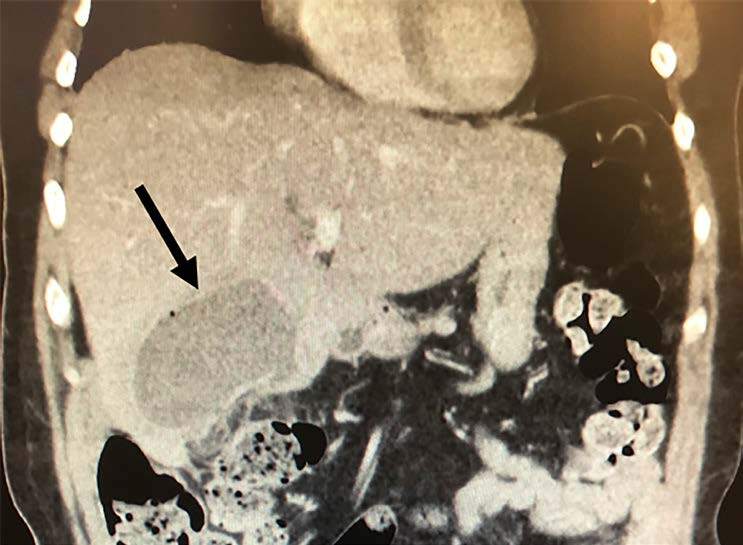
Sagittal view of computed tomography of abdomen/ pelvis with intravenous contrast demonstrating a 7.3 × 5.7 × 7.7 centimeter rim-enhancing fluid collection in the gallbladder fossa, denoted by black arrow.
